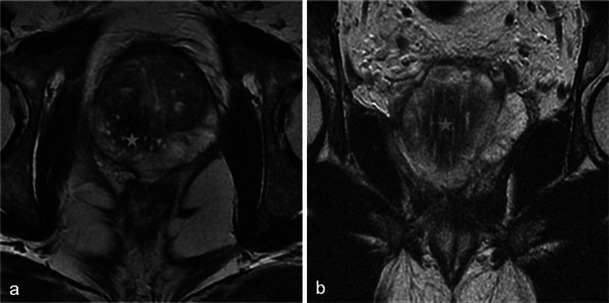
Path of optical fibres. Right prostate lobe treatment for adenocarcinoma Gleason 6 (3 + 3) in a 62-year-old patient with a PSA level of 9 ng/ml. Tumour was not visible on pre-treatment MRI. The paths of the optical fibres in the treated area are visible on the day-7 post-treatment axial T2-weighted image (a) as small hyperintense spots corresponding to thin linear bands (*star*) in the coronal view (b)
